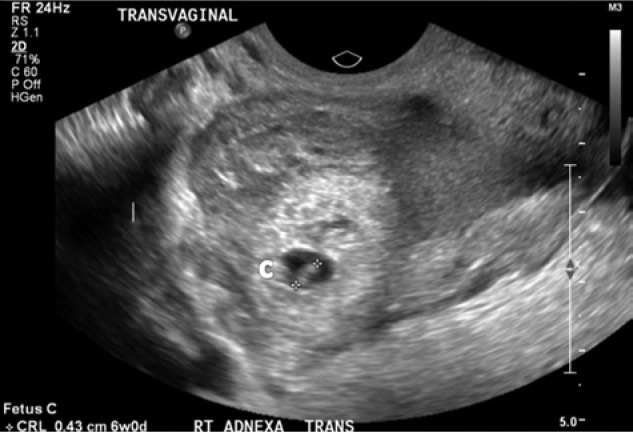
Transvaginal ultrasound of right adnexa showing the right tubal ectopic pregnancy (fetus C) with a gestational age of 6 weeks and cardiac activity.
